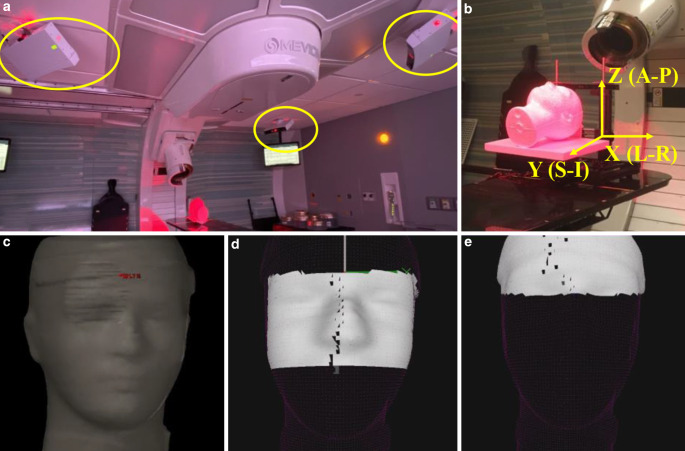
a Optical surface monitoring system (OSMS) setup, which consists of three ceiling camera pods; b each camera projects a red light speckle on the patients; c a reference surface is generated by imposing the body contour from planning CT dataset; and d a region of interest (ROI) is selected and rigidly aligned with the reference surface, with central face selected as the ROI, or e forehead can be selected as the ROI. *L‑R *left-right, *S‑I* superior-inferior, *A‑P* anterior-posterior
